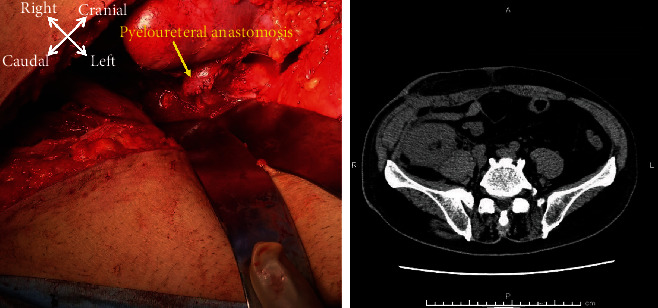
After native nephrectomy, reconstruction is performed with pyeloureteral anastomosis. A running suture is placed in a watertight manner with 3-0 vicryl after placing a double-J ureteral stent. After surgery, computed tomography revealed no residual urine around the graft.
